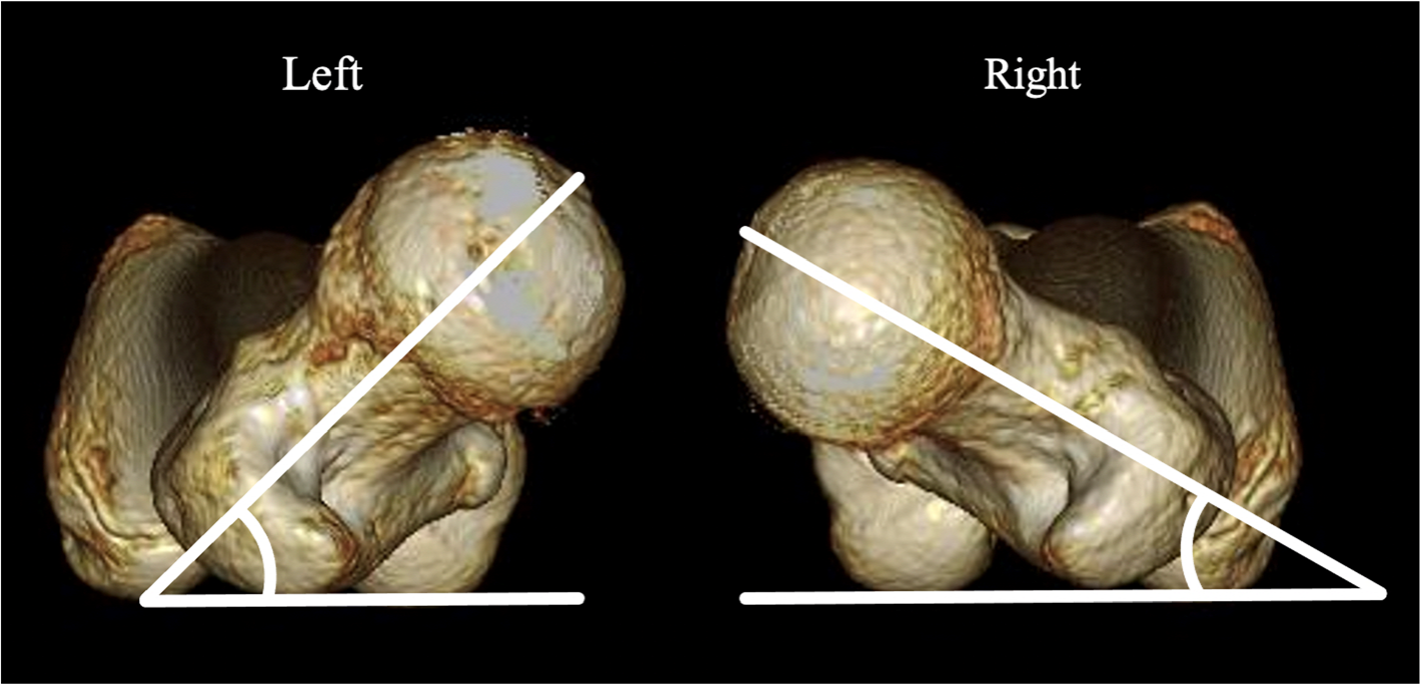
Plain 3D-CT. The anteversion angle of the femoral necks (left/right) was 45/35 degrees. The angle was measured at a point where a tangent on the posterior surface of the medial–lateral femoral condyle overlapped the posterior surface of the greater trochanter when viewing the femur from the cephalic side in the caudal direction
